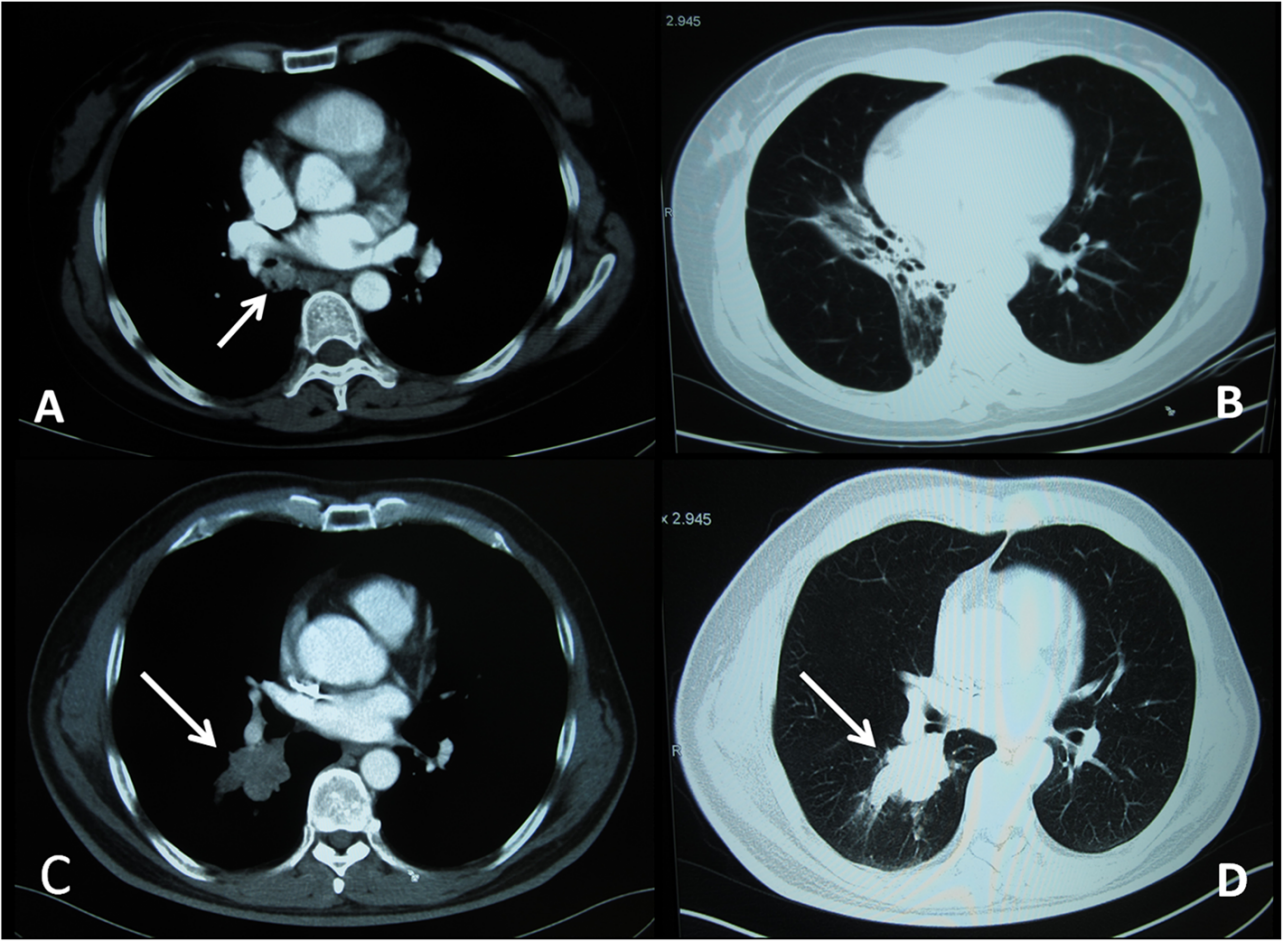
Computed tomography (CT) features. (A) CT showed a mass in the middle segmental bronchus of the right lobe (arrowhead). (B) Patient with partial atelectasis of the right lower lobe was observed. (C and D) CT revealed a mass shadow in the hilus of the right lung of the same patient (arrowhead). CT, computed tomography.
